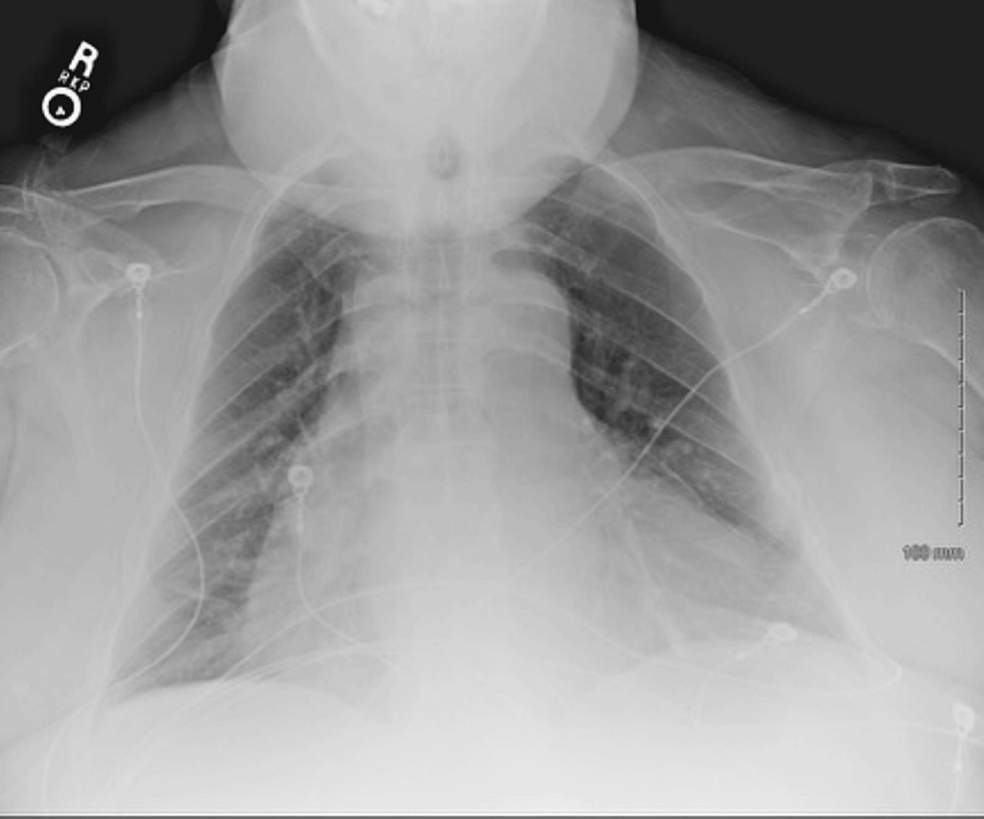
Chest radiograph AP view showing no acute cardiopulmonary findings associated with cardiomegaly. AP, Anterior-posterior
